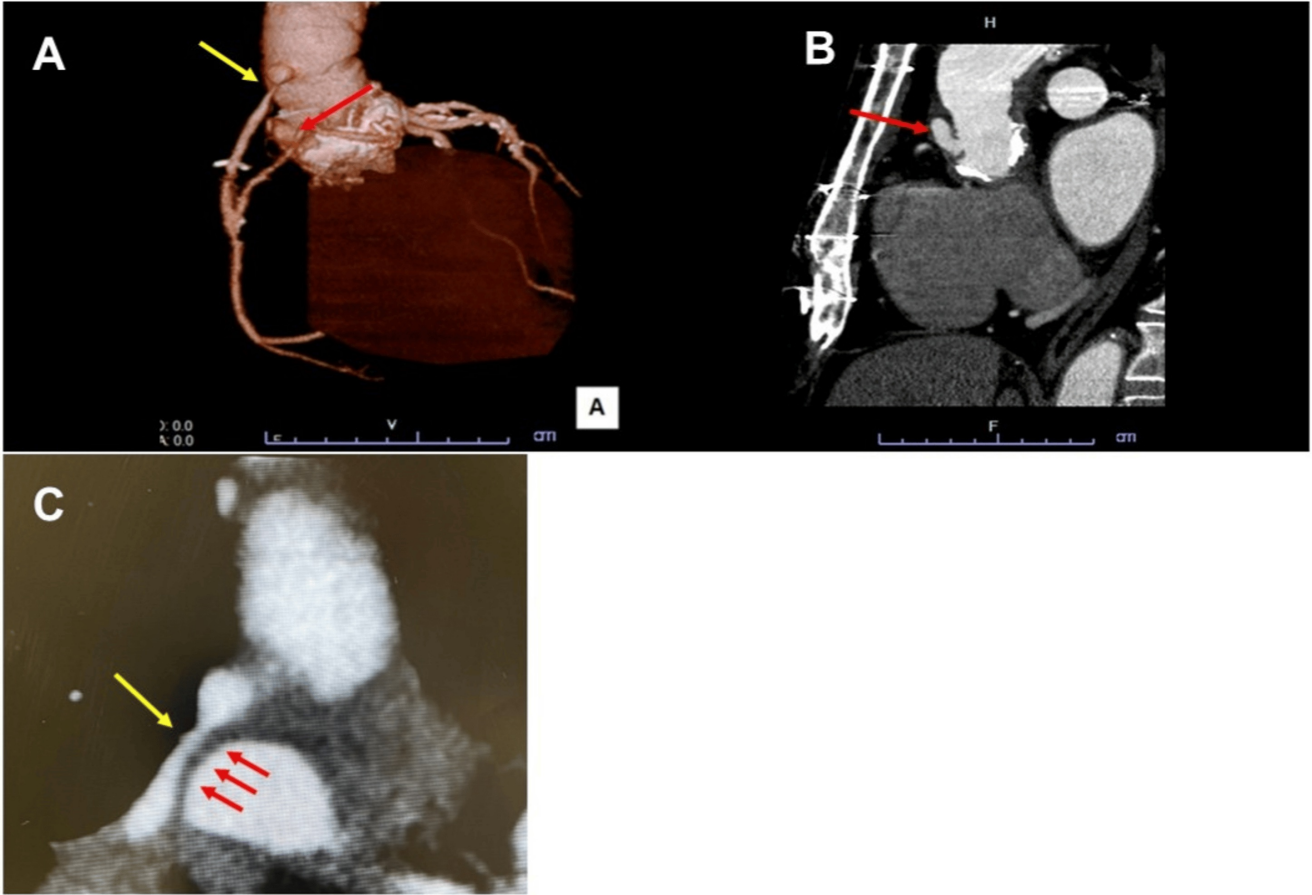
(A, B) CT scans showing an ascending aortic pseudoaneurysm (red arrow) with a neck diameter of 7 mm and associated SVG stenosis (yellow arrow). (C) CT scan showing the pseudoaneurysm (red arrow) compressing the SVG (yellow arrow); the pseudoaneurysm measured 20 × 10 mm. CT: computed tomography, SVG: saphenous vein graft
